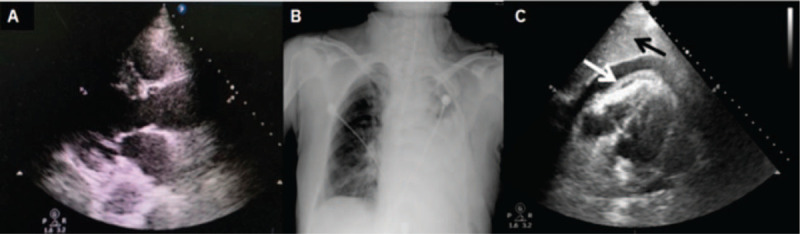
No evidence of pericardial effusion was revealed by transthoracic echocardiography (TTE) before left pneumonectomy surgery (A). Left large pleural effusion was revealed by chest X-ray four days after surgery (B). Large pericardial effusion and suspected right ventricular collapse (white arrow) were revealed by TTE on the subxiphoid four-chamber view, in which the liver (black arrow) was observed to be located between the probe and the heart (C). TTE = transthoracic echocardiography.
